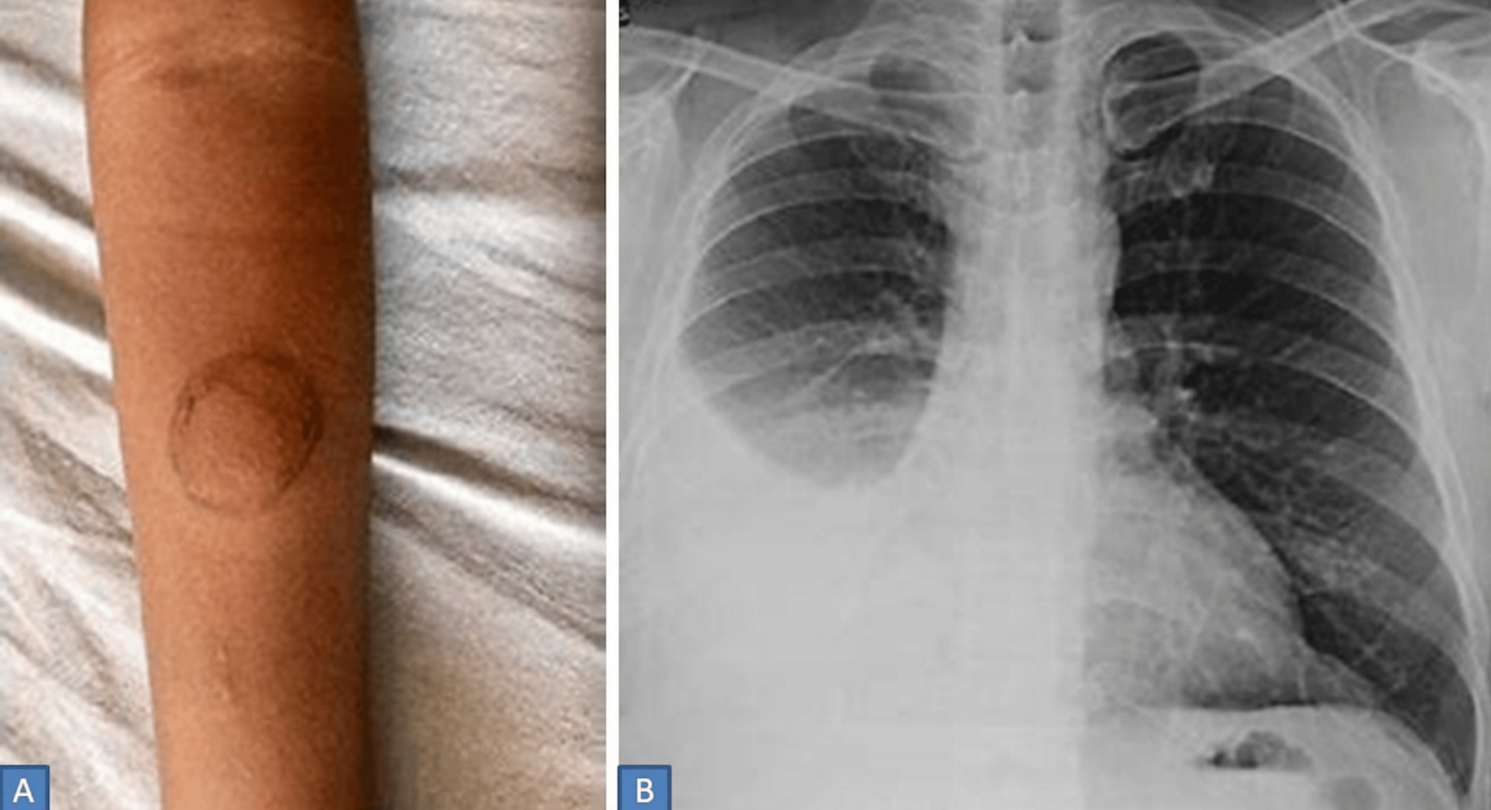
Clinico-radiological images. Patient showing positive Mantoux test with induration of 22 mm² (A). Chest X-ray Postero-anterior view showing right-sided pleural effusion with otherwise normal parenchyma (B).
